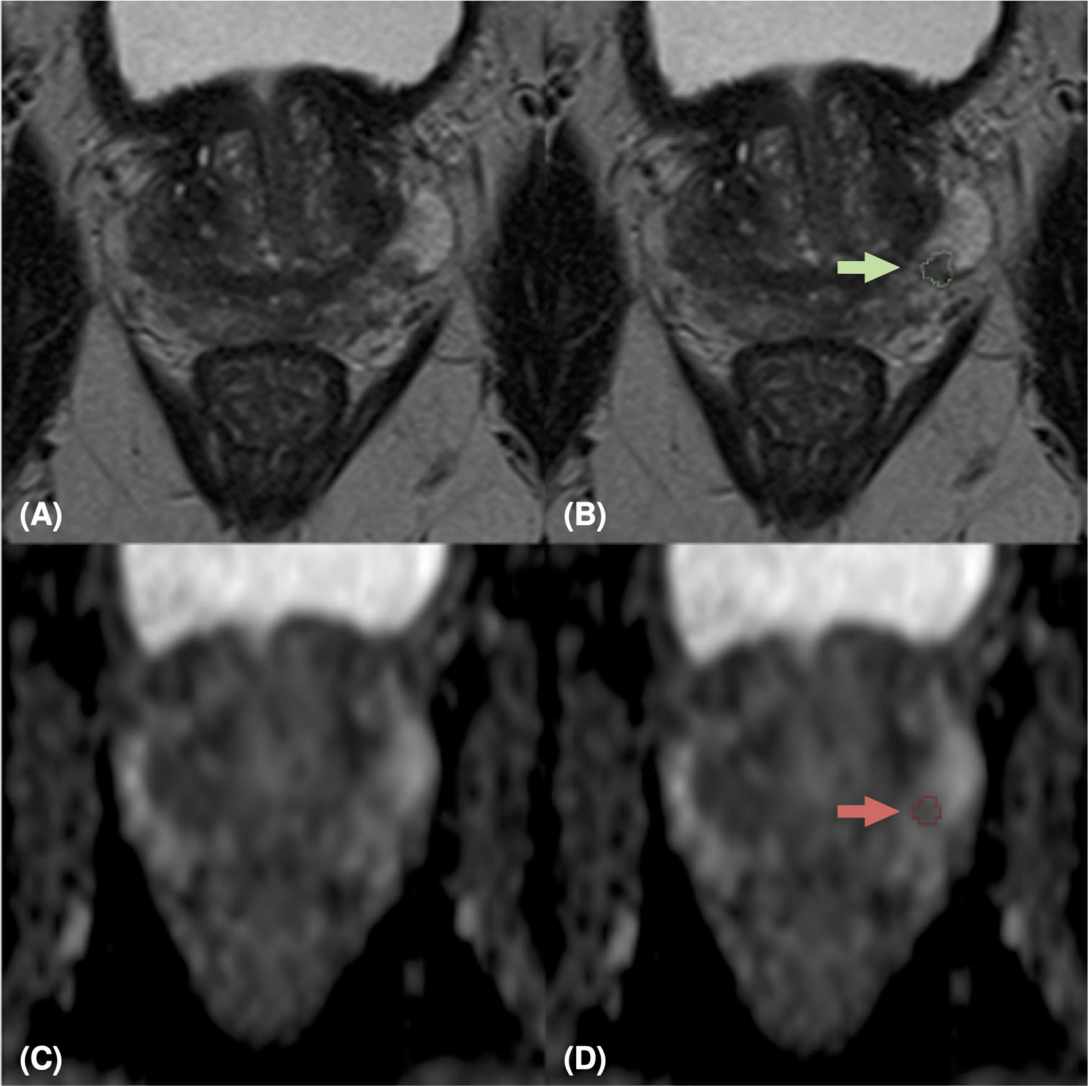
MpMRI of a 65-years old patient with indeterminate mpMRI result (PI-RADS=3), PSA=5.49 ng/ml, GS=3+4 (ISUP 2). MRI zoomed images containing the target lesion, respectively axial T2-weighted image (A), ADC map (C), and their relative lesion segmentations (B, D). The green (B) and red (D) arrows point out the segmented lesion in T2 and ADC images, respectively.
